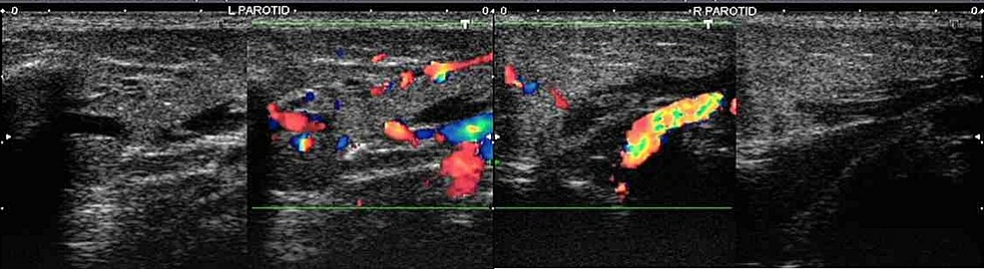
Ultrasound greyscale images showing diffuse hypoechogenicity and enlargement of the left and right parotid glands, and color Doppler images demonstrating increased vascularity (left>right) within parotid glands suggestive of acute parotitis
